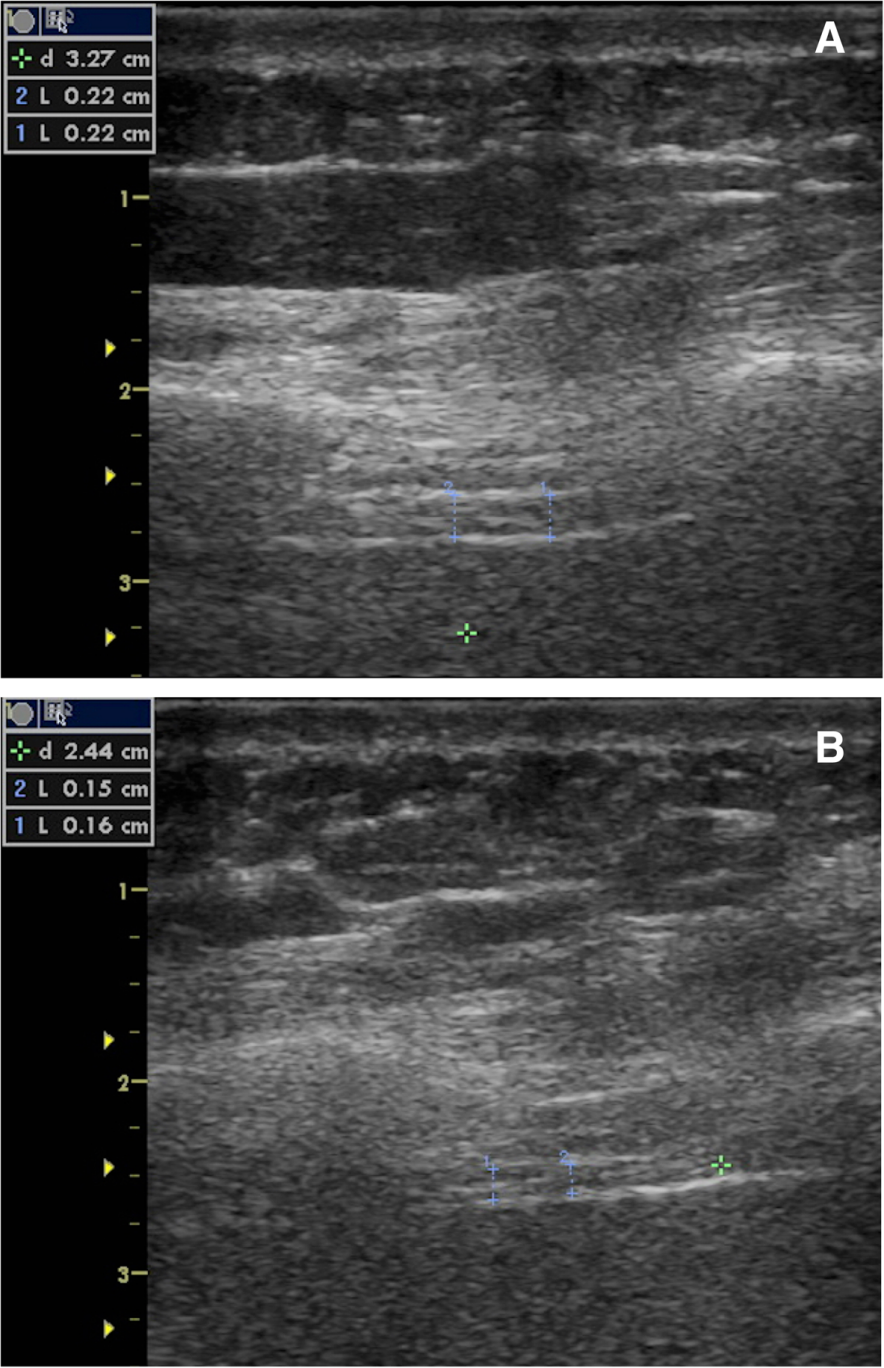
Ultrasound sample images. Right hemidiaphragm thickness recording image after admission (a) and on day 5 (b) as obtained during the study
